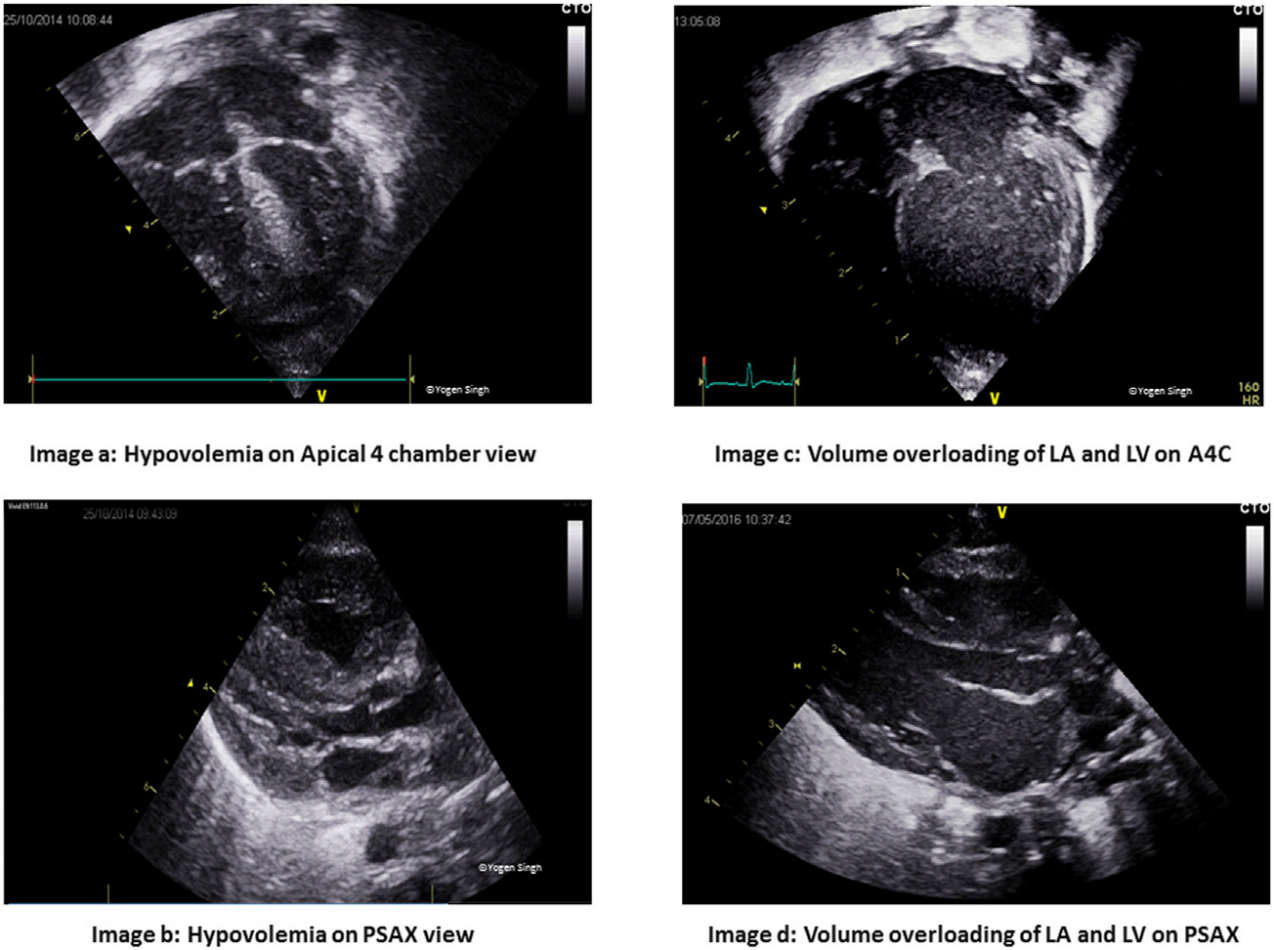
Assessment on cardiac filling on visual inspection “eyeballing.” Images (A,B) show under-filled heart in apical 4 chamber (A4C) and parasternal long axis (PLAX) views. Images (C,D) show volume overloading of left atrium (LA) and left ventricle (LV) in A4C and PLAX views.
